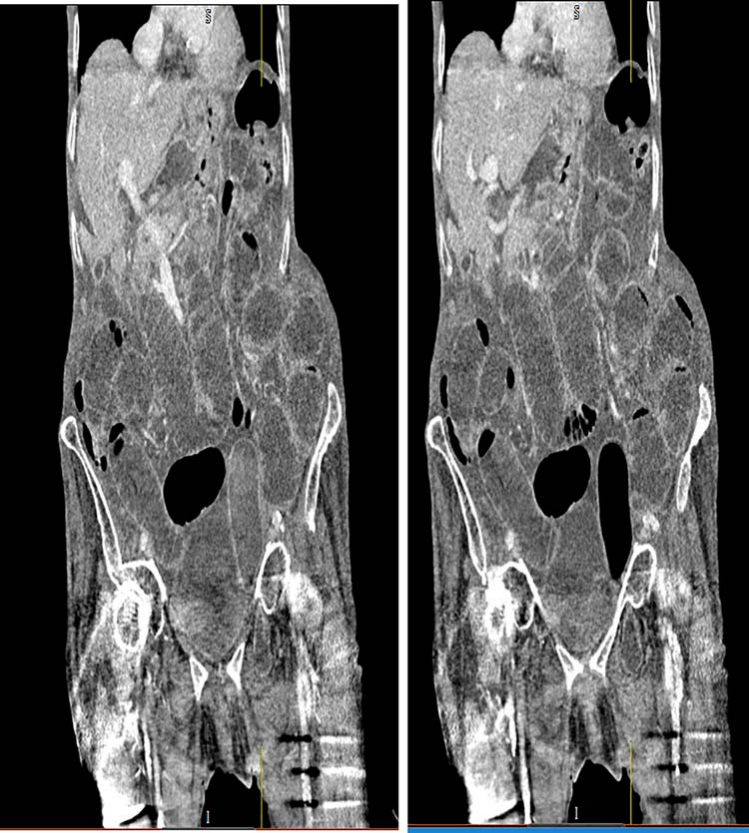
Coronal view CT scan with IV contrast showing dilated small bowel loops and bowel loop through the left obturator canal.
